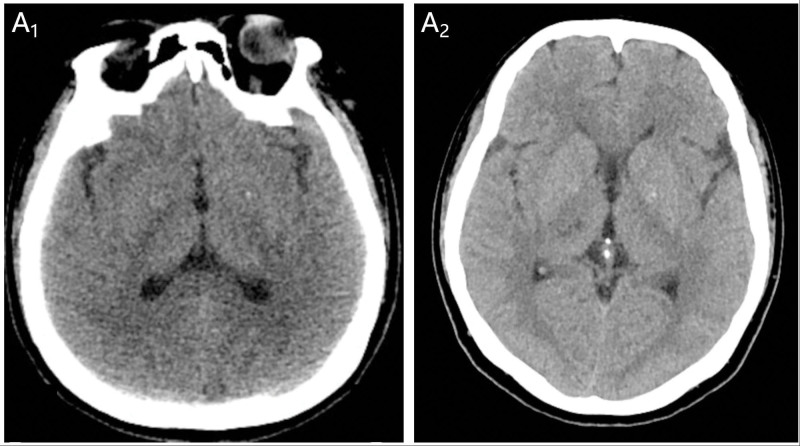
Computed tomography (CT) images during thrombolytic therapy with alteplase. (A_1_) On admission, CT of the head was normal. (A_2_) Twenty-four hours after the end of intravenous thrombolysis, the reexamination of the head CT showed no hemorrhage.
